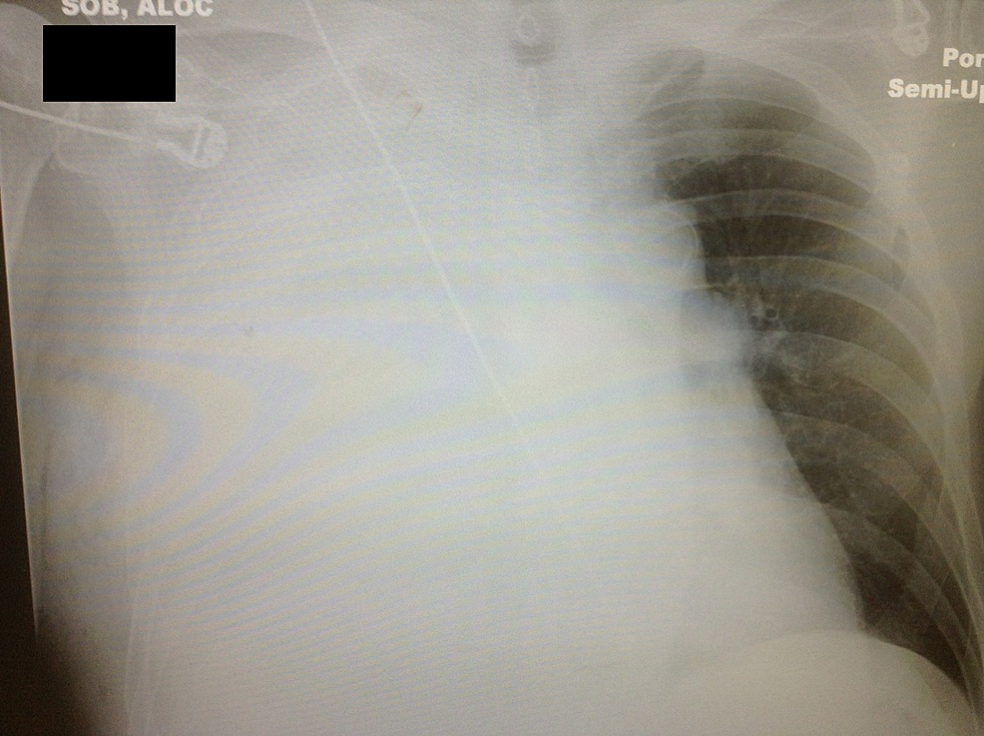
Chest X-ray at the time of arrival.
